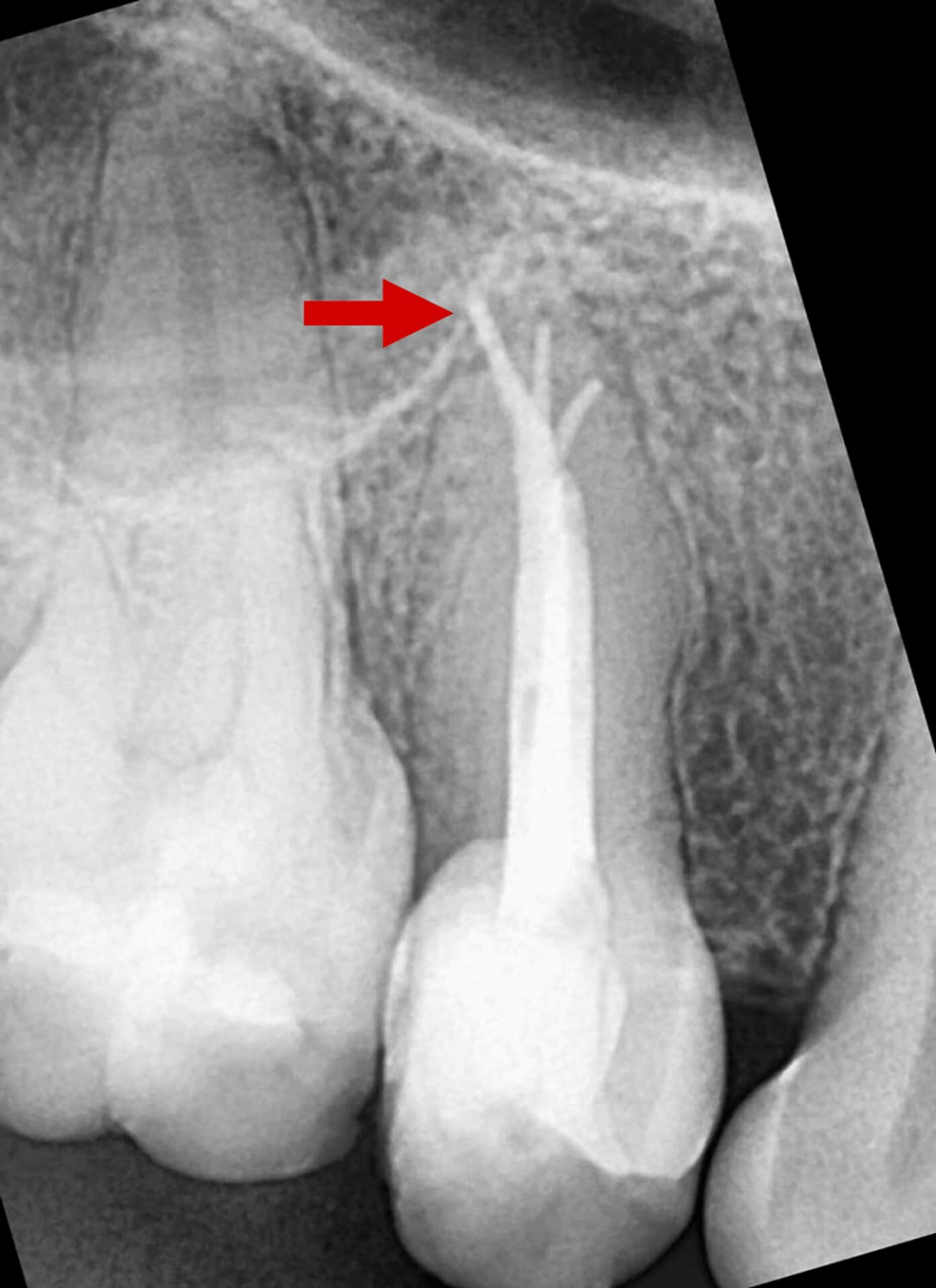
Post-endodontic restoration radiograph Arrow depicting distobuccal canal
